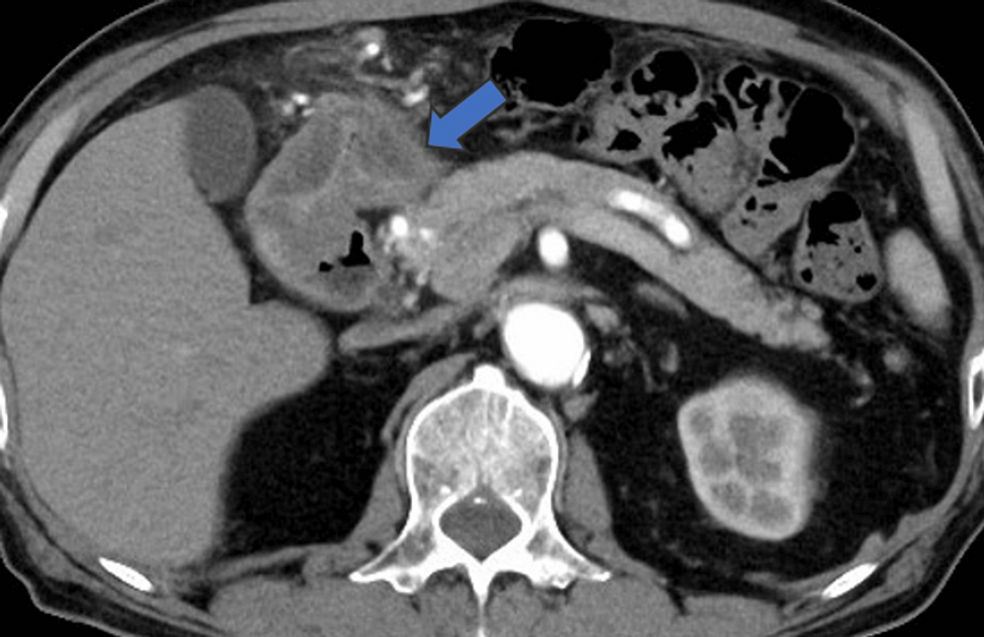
Contrast-enhanced CT Contrast-enhanced CT of the transverse section. Focal edematous wall thickening was seen in the antral measurement of the gastric body (arrow). CT, computed tomography
